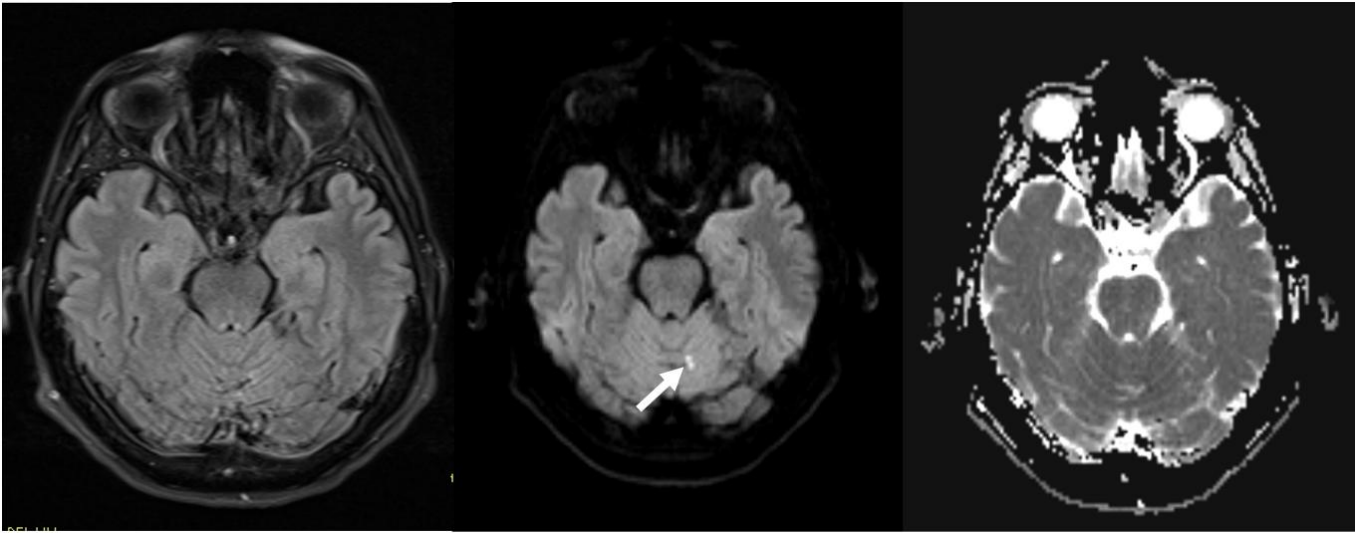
Right to left: Fluid-attenuated inversion recovery (FLAIR T2), diffusion-weighted imaging (DWI), apparent diffusion coefficient (ADC). Diffusion-restricted focus in the left paravermian region of the cerebellum, also seen on FLAIR sequence; there was another similar one in the left frontoparietal cortex (not shown). These findings were consistent with acute lacunar infarcts.
